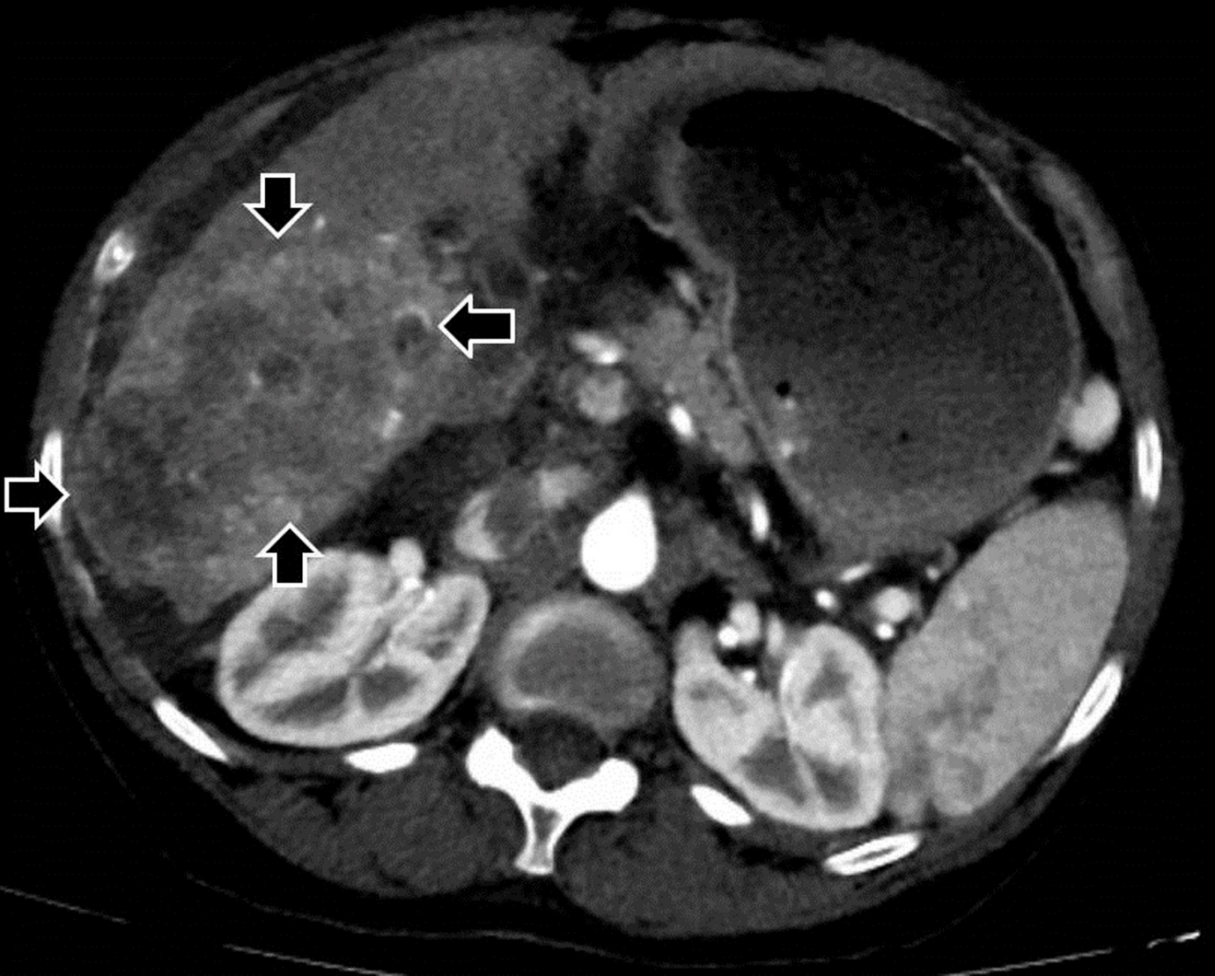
Computed tomography image of the liver with contrast showing a large, about 10 x 6 cm-sized right hepatic mass (black bold arrows).
